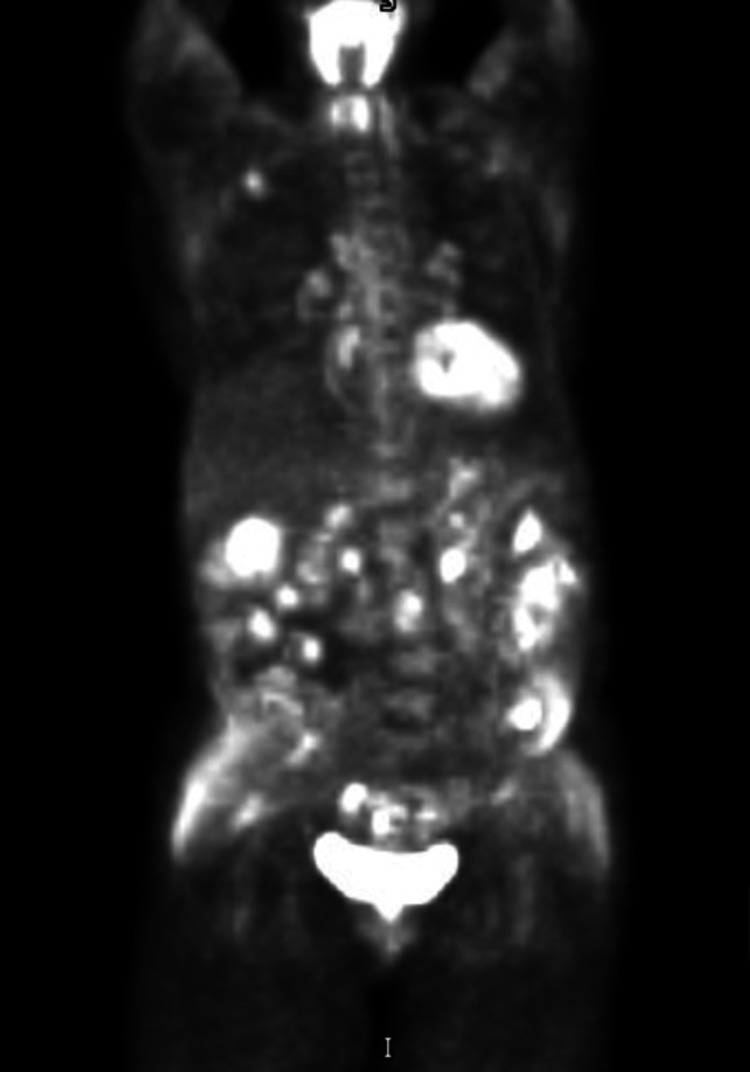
Positron emission tomography/computed tomography scan.
